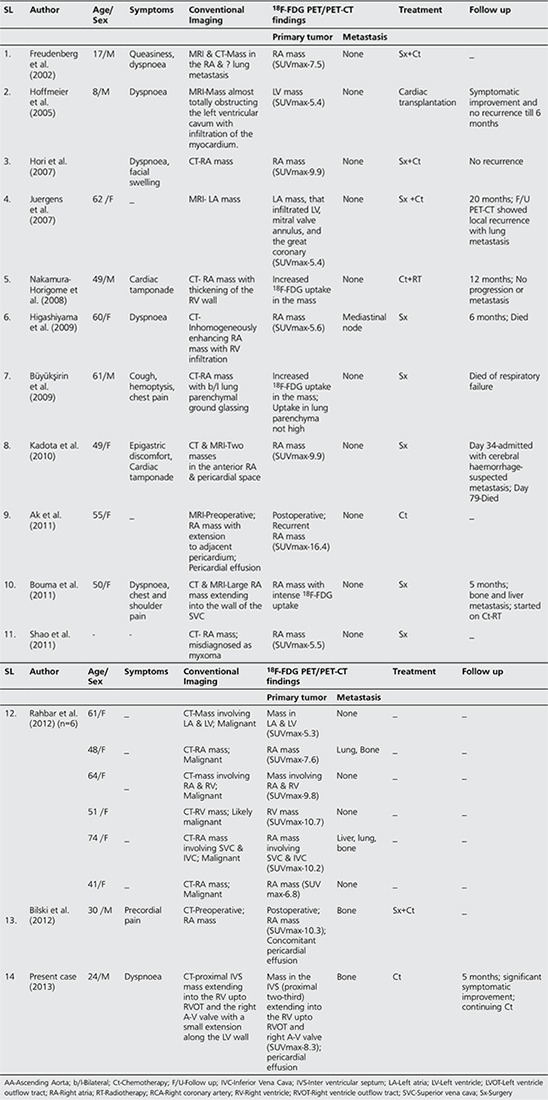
Review of literature-18F-FDG PET/PET-CT for primary cardiac angiosarcoma reported in the English literature
